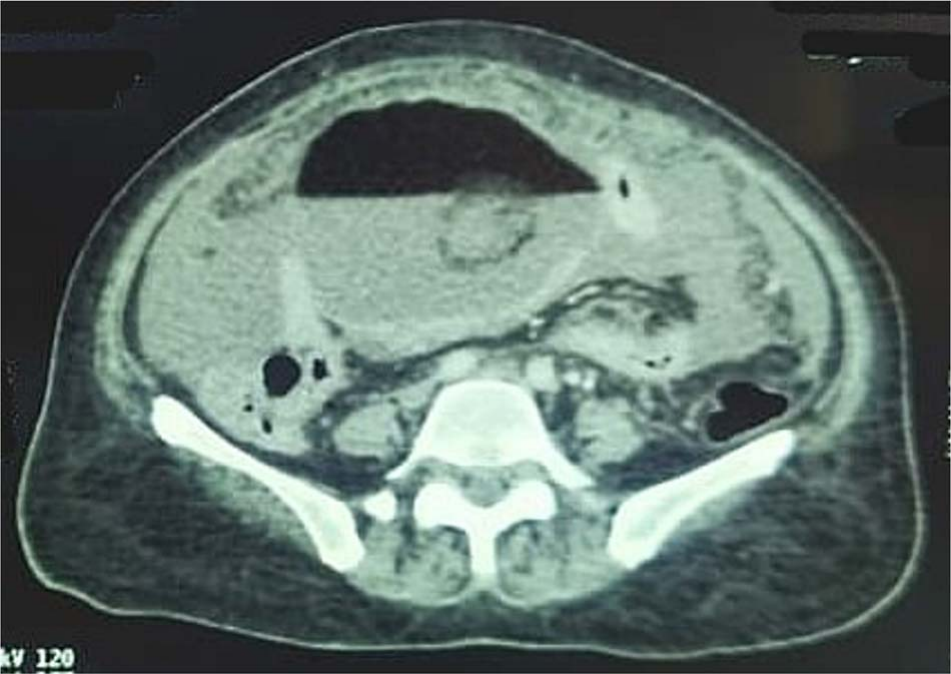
CT image showing a cystic ovarian mass with fatty and fluid components, calcification, and a Rokitansky nodule.
